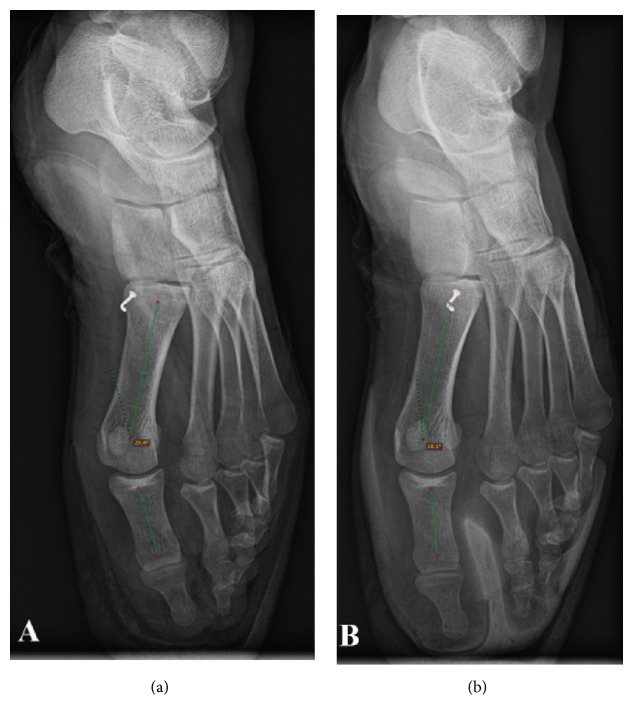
X-ray example: toe deviation angle with standard toe cap (a) and with customized toe cap (b).
